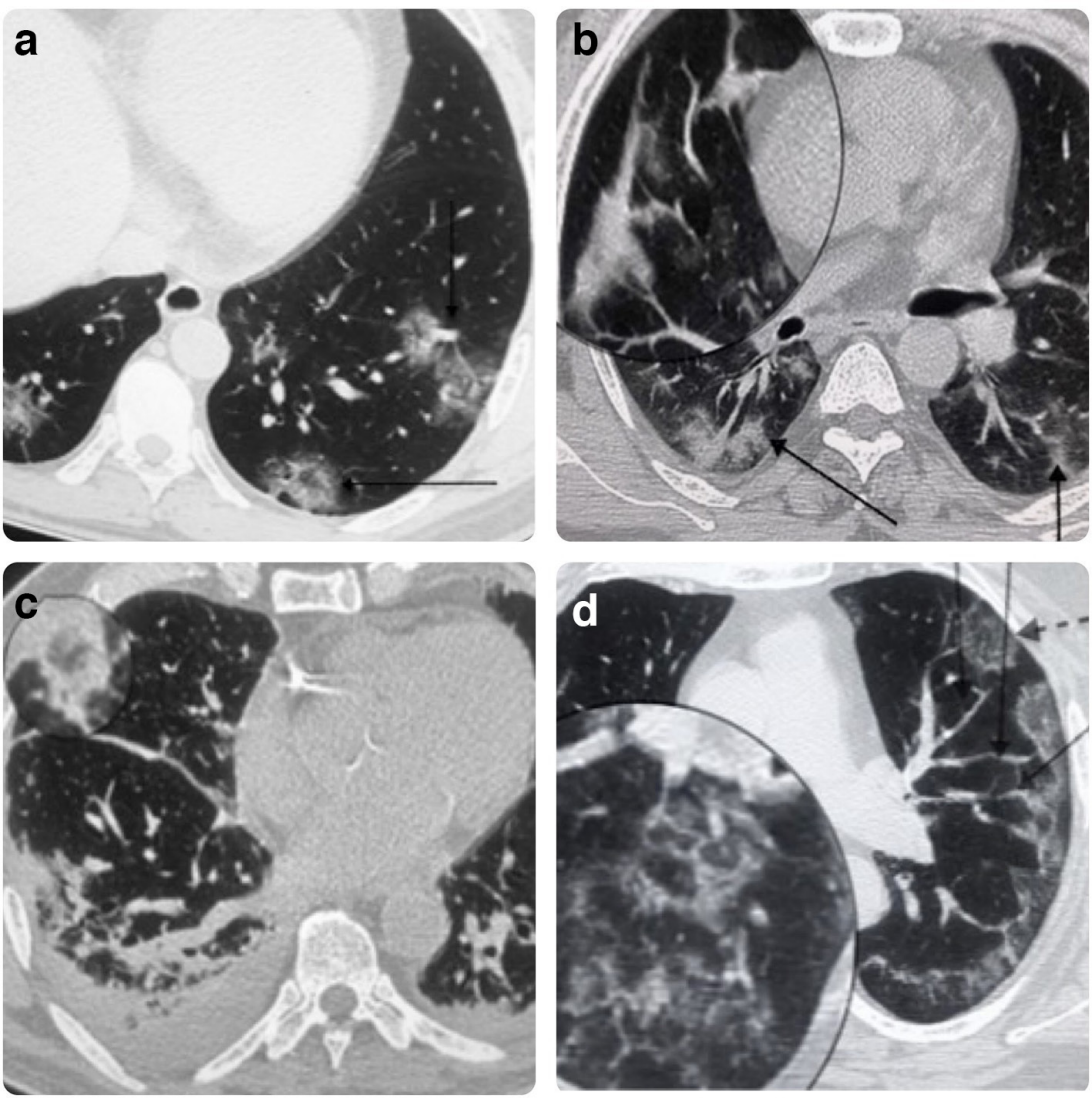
A: Low-dose CT chest shows bilateral rounded GGO in the lower lobes. The vertical arrow points to the vessel engorgement, and the horizontal arrow points to the Reverse Halo sign. B: Chest CT lung window, the magnified section shows the subpleural bands, vertical arrow points to consolidation with GGO, and the inclined arrow points to an area of predominant consolidation. C: Chest CT lung window, the magnified section shows the Reverse halo sign. There is bilateral mild pleural effusion with area of predominant consolidation.D: Axial Section Lung window shows, rounded and patchy peripheral GGO. The interrupted arrow points to rounded shaped GGO. The solid arrows point to vessel engorgement. The magnified section shows the crazy paving pattern. GGO, ground glass opacity.
